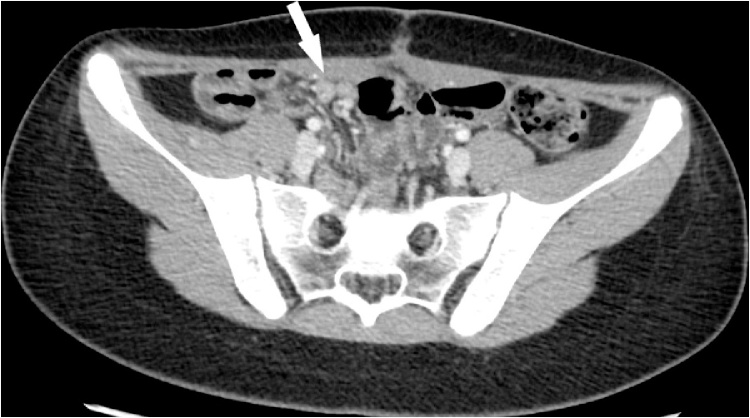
Pre-operative CT-SCAN: peritoneal metastasis were found in the contest of the right rectus muscle and of the mesentery next to the umbilicus’’.
